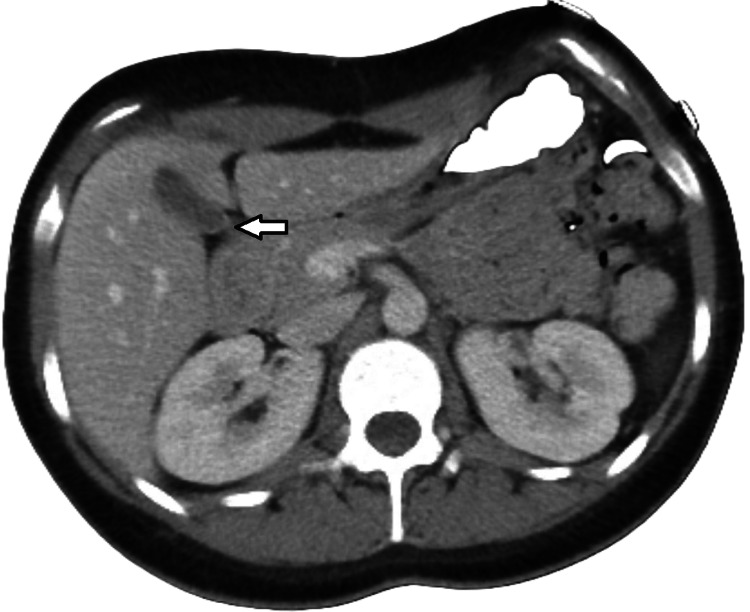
Computed tomography (CT) abdomen and pelvis with intravenous contrast Computed tomography (CT) abdomen and pelvis with intravenous contrast shows a normal gallbladder without stones or dilatation.
